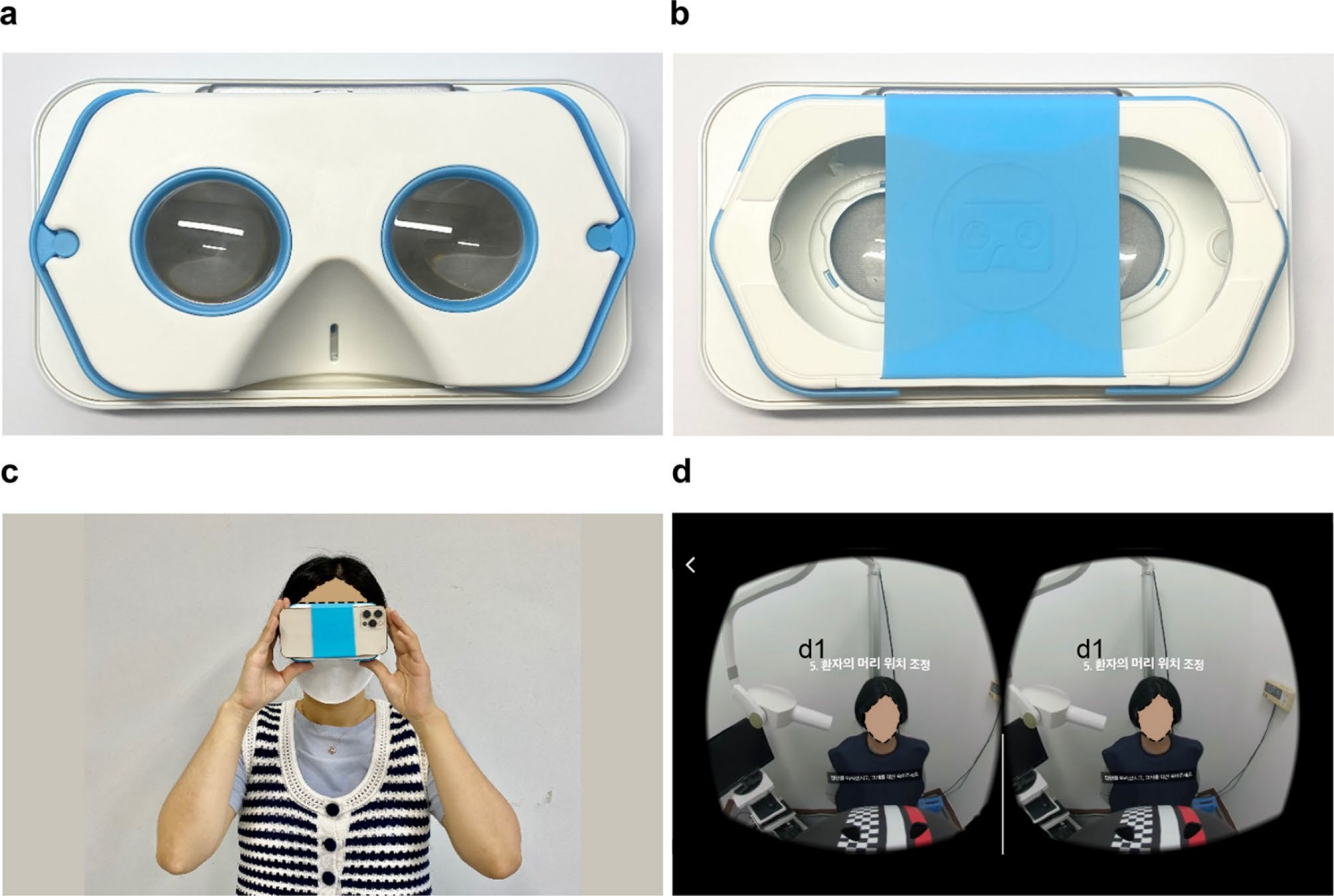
Dental radiography learning tool based on virtual reality. (a) Anterior aspect of prefabricated head-mounted display (pHMD). (b) Posterior aspect of pHMD. (c) The learner attaches a smartphone to pHMD and uses it as a head-mounted display. (d): This photo shows that a 360° recorded video is played by dividing the same video into the left and right eyes on a smartphone attached to pHMD. d1: The sentence “d1” stands for “guide and adjust the patient’s head appropriately for dental radiography” in Korean
